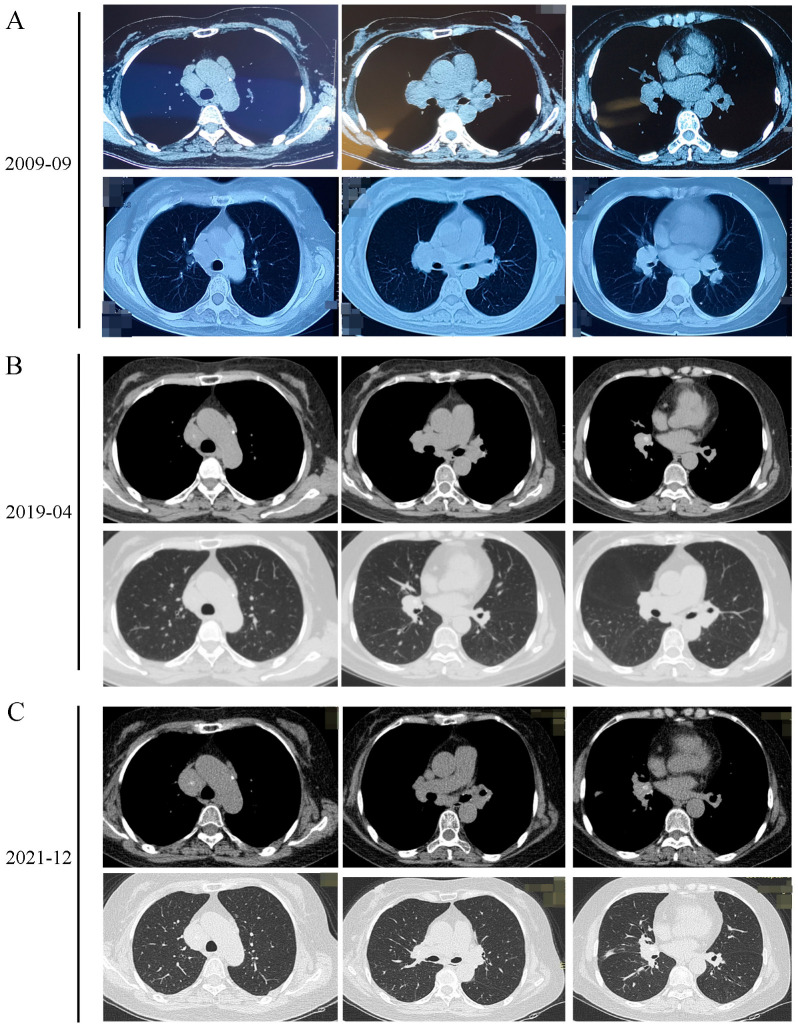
(A) The enlargement of intrathoracic lymph nodes was detected by chest CT scans in 19 September 2009. (B) 3 April 2019. (C) 2 December 2021. A new calcification of the mediastinal lymph nodes and a new opacity in the right middle lobe of the lung appeared in 3 April 2019 and 2 December 2021.
